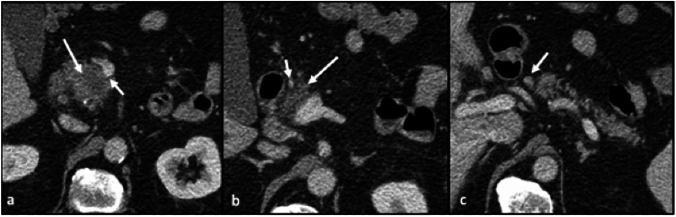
Axial CT sections with contrast in the portal venous phase. a Hypoenhancing mass in the anterior pancreatic head (long arrow), with slight flattening and deformation of the SMV (short arrow). b Disease infiltrates superiorly into the dorsal mesoduodenum (long arrow), demarcated by the gastroduodenal artery (arrow). c Enlarged infrapyloric node, indicating early involvement of disease spread (arrow)
